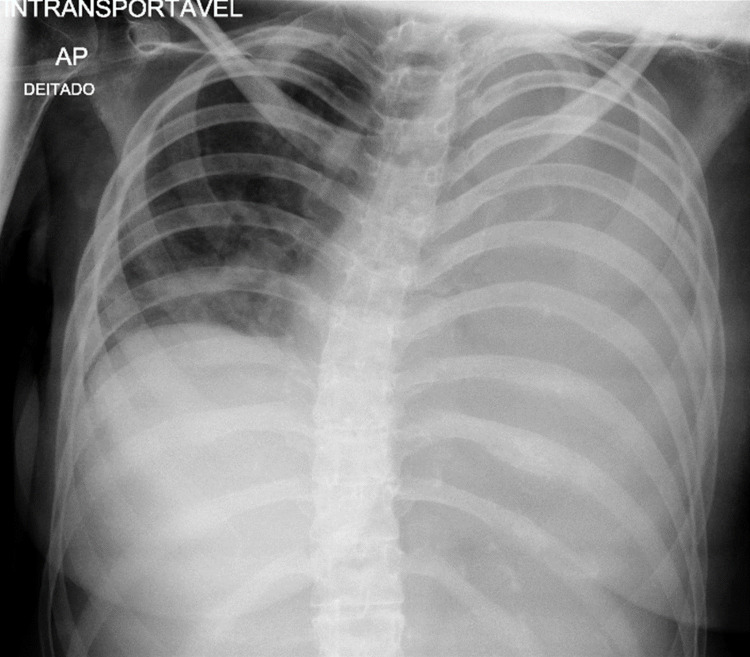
Chest X-ray showing an opacified left hemithorax.
